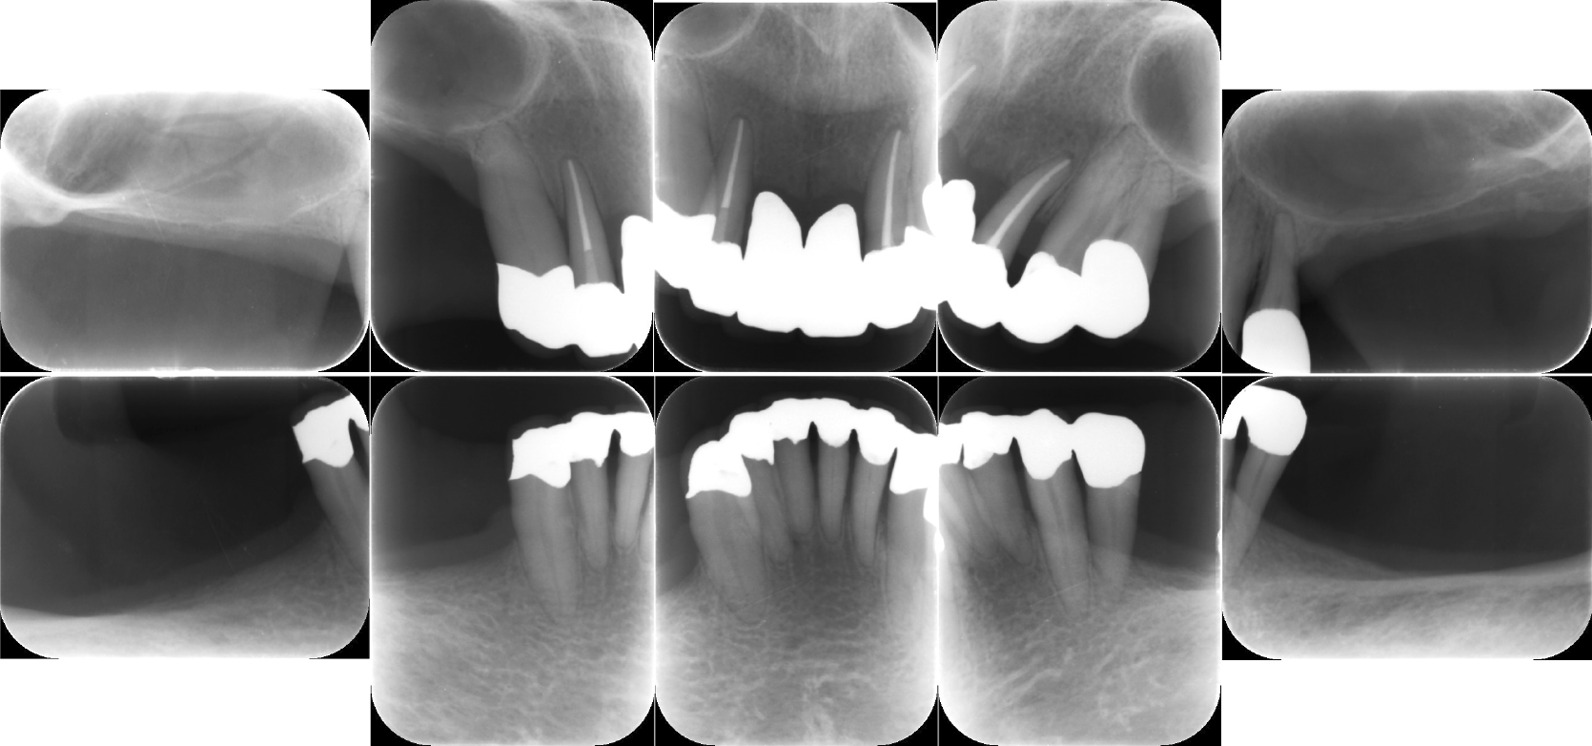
Periapical radiography reveals no sign of progression of bone resorption
